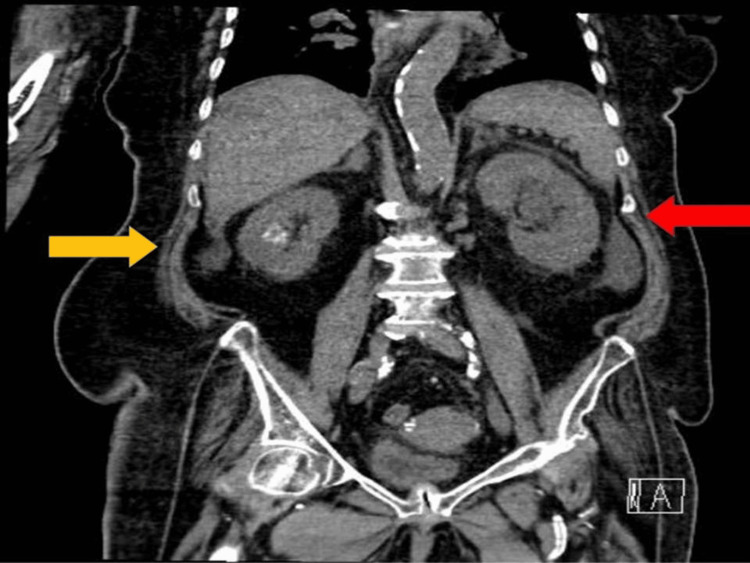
Non-contrast CT image showing bilateral hydronephrosis, more prominent on the left side. Yellow arrow: right kidney showing mild fullness; Red arrow: left kidney showing mild hydronephrosis on non-contrast CT.
